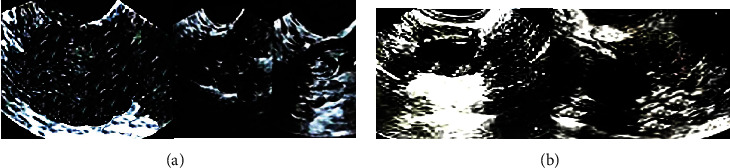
Ultrasound data of a patient before and after treatment (24 years old, married). A was the transvaginal color Doppler ultrasound images before treatment; B showed the transvaginal color Doppler ultrasound images after treatment.
